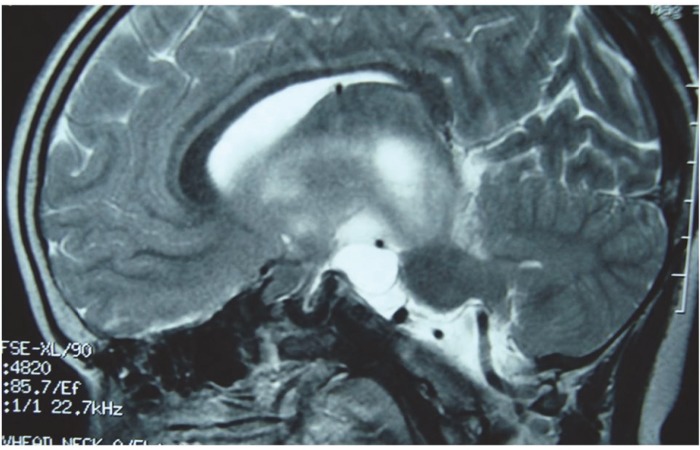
Sagittal section of MRI brain scan shows large mass in optic chiasmatic region suggestive of optic pathway glioma with hamartomas
